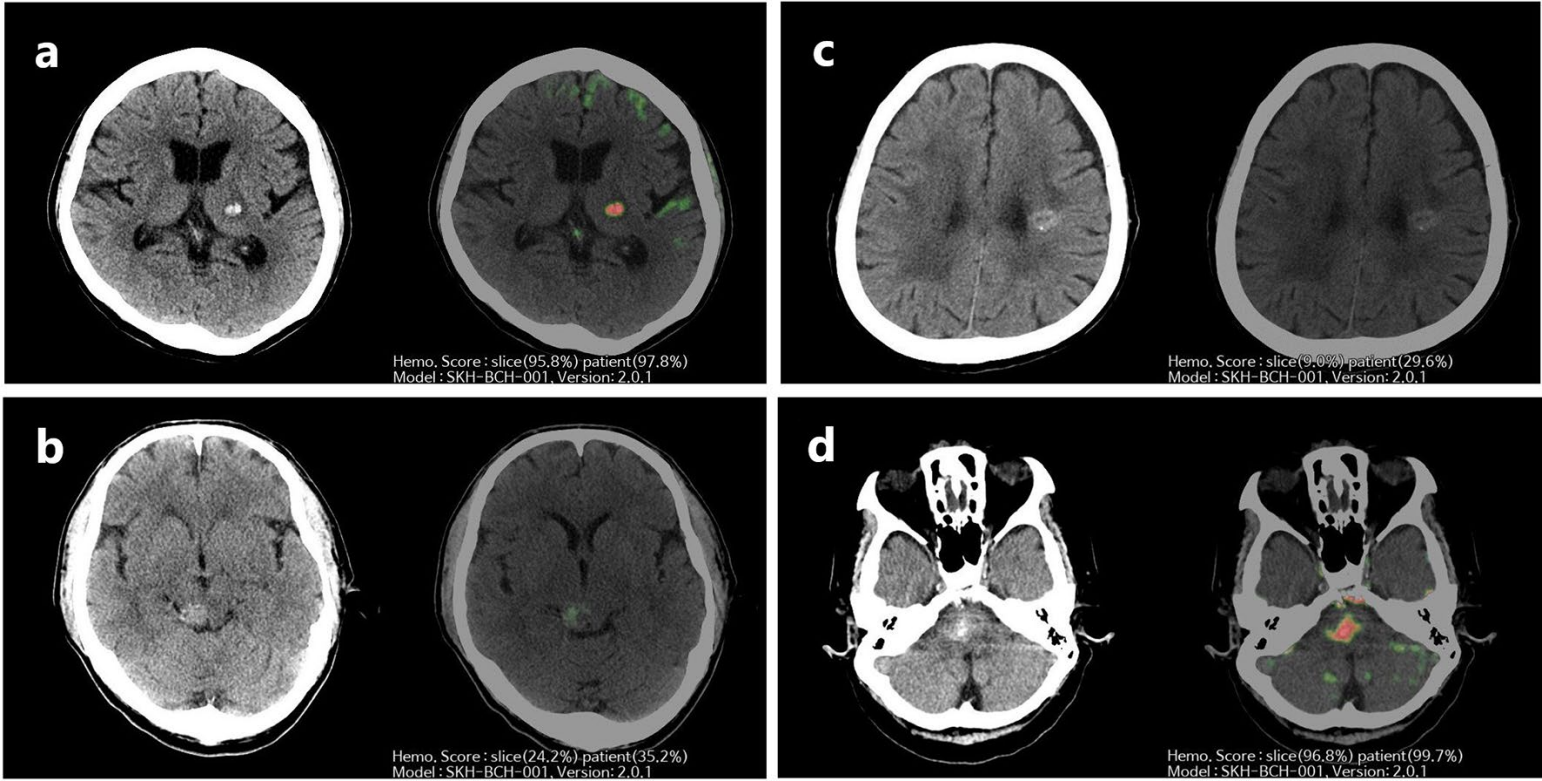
Representative images of the AI algorithm for differentiating CCM and AIH. AI, artificial intelligence; CCM, cerebral cavernous malformation; AIH, acute intraparenchymal hemorrhage; CT, computed tomography. (a) Brain CT shows AIH in the left thalamus. AI-assisted brain CT analysis provided probability scores of hemorrhage in a slicewise (95.8%) and patientwise (97.8%) manner. All six reviewers correctly interpreted the images as AIH for both AI-unassisted and AI-assisted interpretations. (b) Brain CT shows a CCM on the right side of the midbrain. AI-assisted brain CT analysis provided a probability score of hemorrhage in a slicewise (24.2%) and patientwise (35.2%) manner. One radiology resident and one emergency department physician incorrectly interpreted the images as AIH in the AI-unassisted brain CT. However, with the use of AI assistance, all reveiwers correctly diagnosed the images as CCM. (c) Brain CT shows a CCM in the left corona radiata. AI-assisted brain CT analysis generated probability scores of hemorrhage in a slicewise (9.0%) and patientwise (29.6%) manner. All six reviewers accurately identified the images as CCM for both AI-unassisted and AI-assisted interpretations. (d) Brain CT shows AIH in the pons. AI-assisted brain CT analysis generated a probability score of hemorrhage in a slicewise (96.8%) and patientwise (99.7%) manner. All neuroradiologists and emergency department physicians erroneously interpreted the images as CCM in the AI-unassisted brain CT. Nevertheless, with the use of AI assistance, all reviewers accurately diagnosed the images as AIH.
